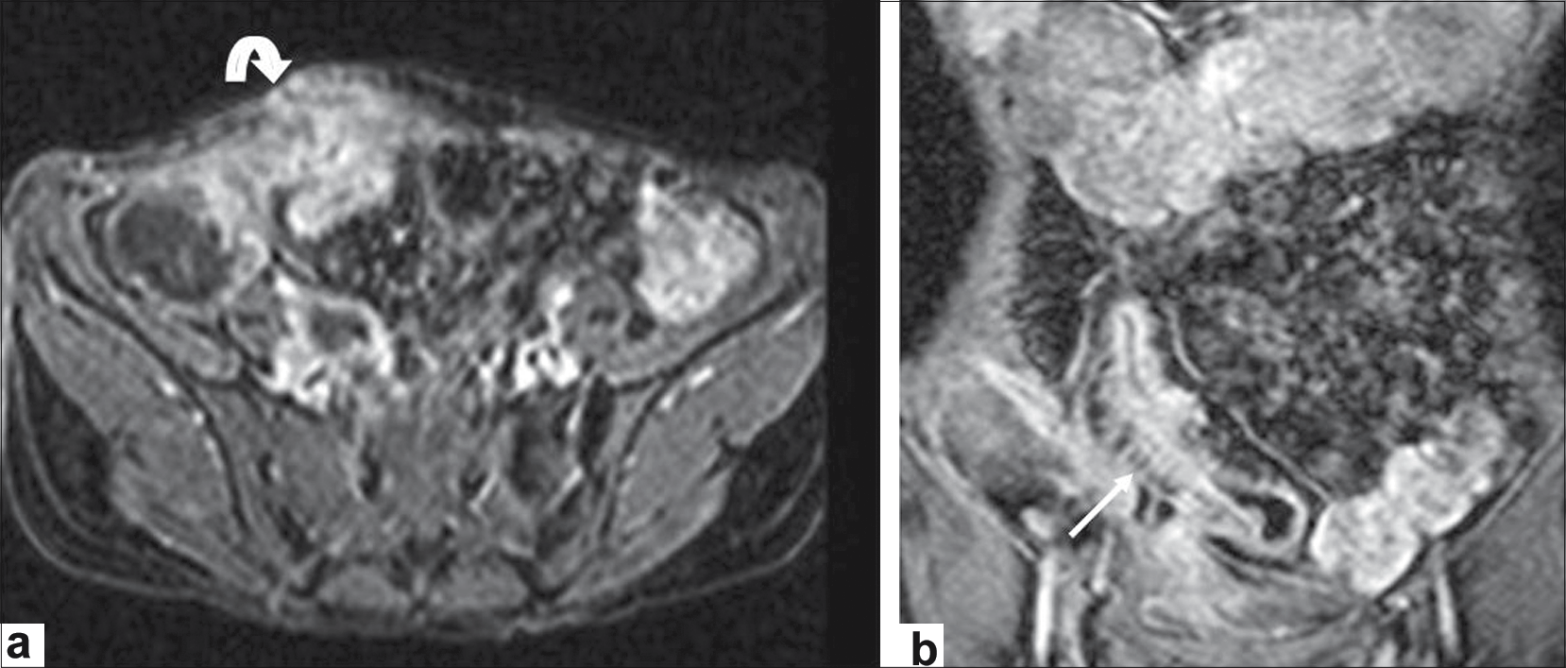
Axial and coronal MR images show (a) diffuse wall thickening and (b) increased gadolinium enhancement of the terminal ileum with fistulas between terminal ileum-bladder (straight arrow) and abdominal wall (curved arrow in a)
